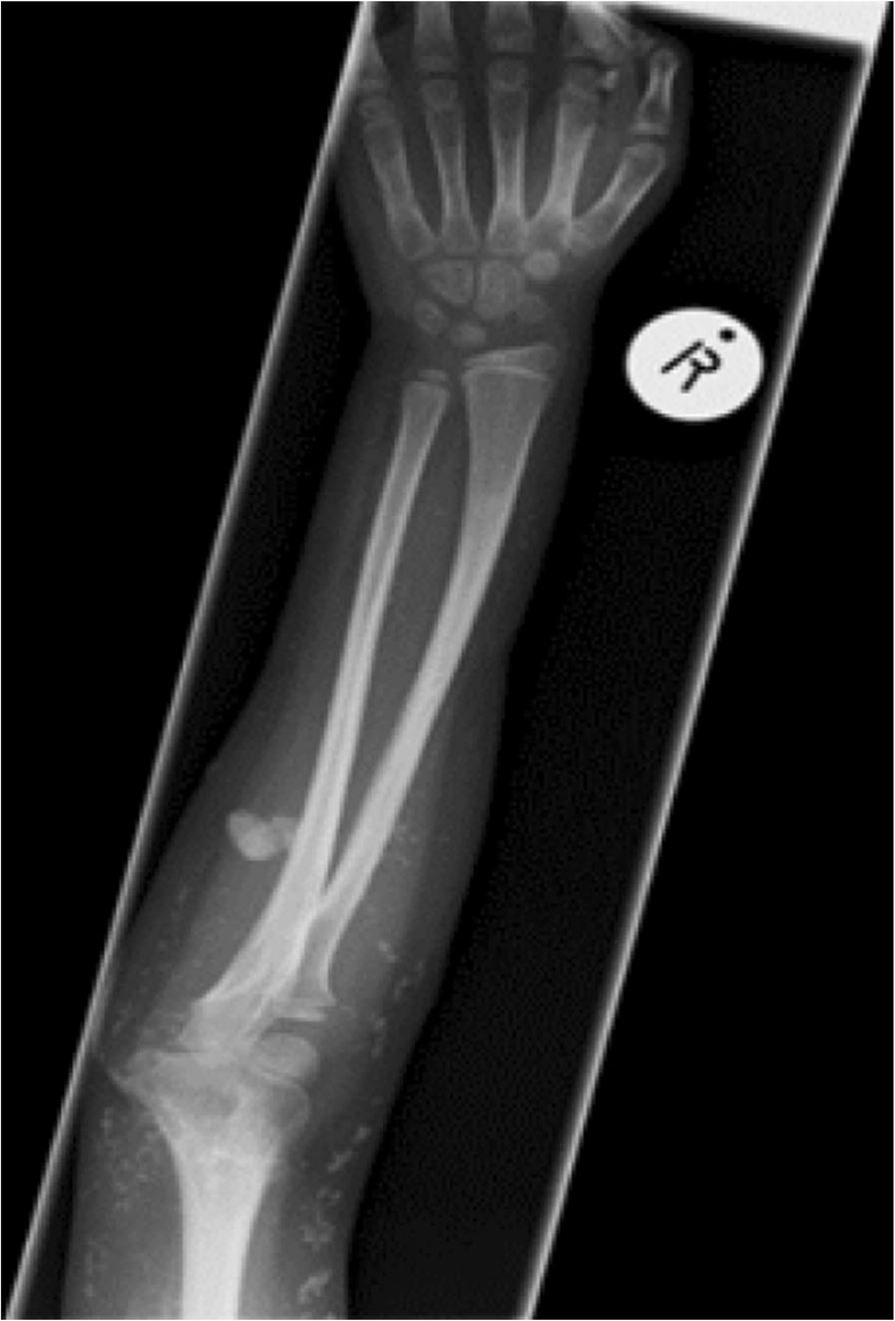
Forearm radiograph showing mixed tumoural and planar calcinosis in one of the JDM patients
